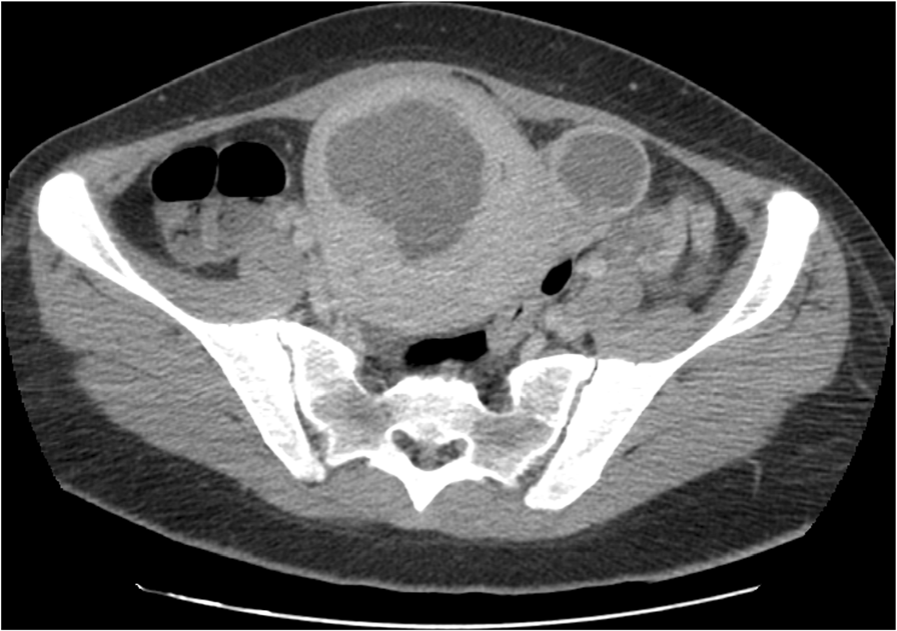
CT scan, abdominal view: mild bilateral hydroureteronephrosis and enlarged uterus with heterogeneous masses.
